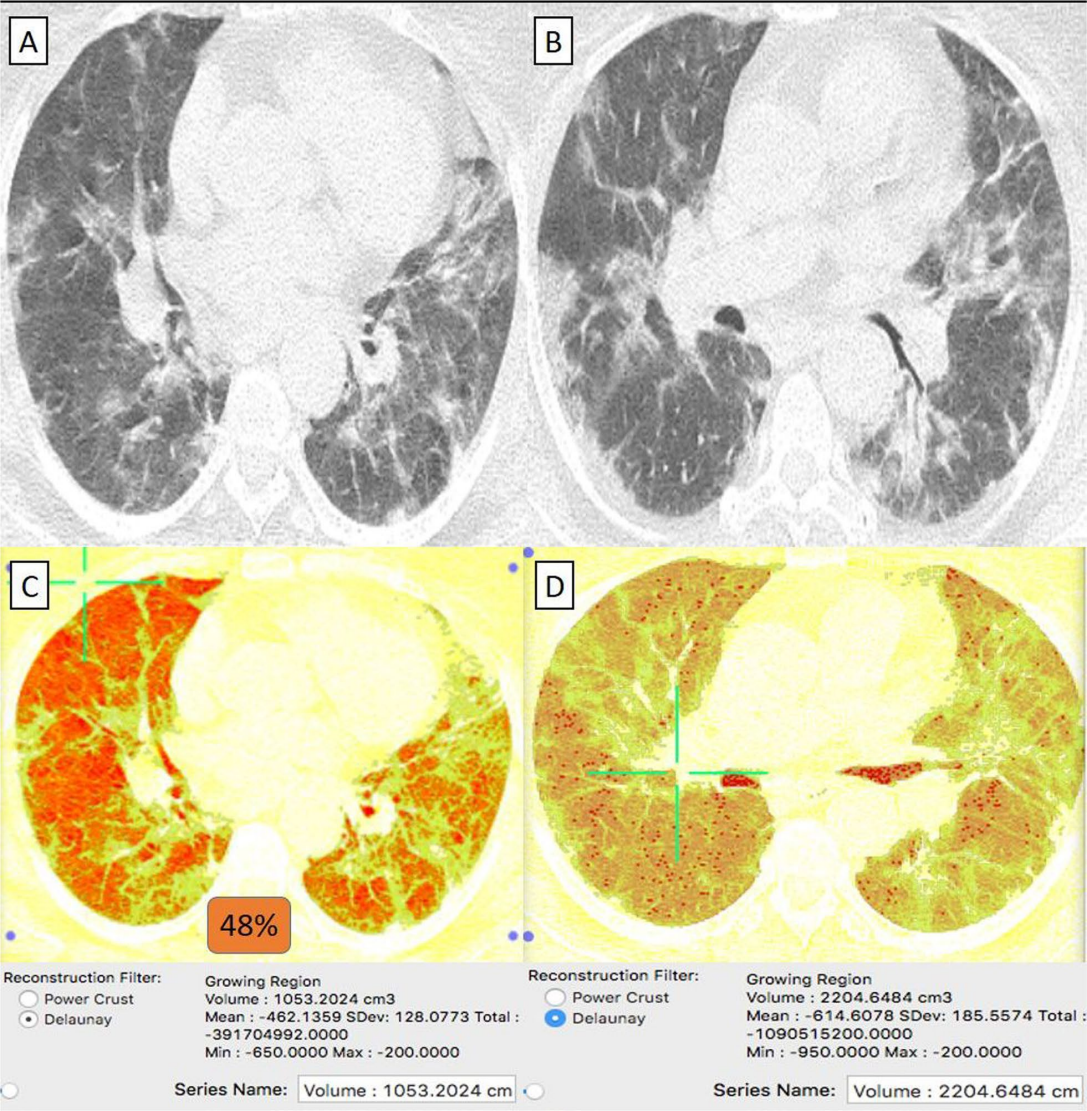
A 69-year-old female cardiac and diabetic patient who had received “Sinopharm” vaccine then got COVID-19 infection. A, B Axial chest CT cuts (lung window) showed bilateral mainly peripheral located and to lesser extent bronchocentric ground-glass pneumonic patches. C CT-Volumetry of the pathological lung parts averages 1053 cc. D CT-Volumetry of total lung volume averages 2204 cc. So, 48% of the lung parenchyma was diseased (Score 2)
